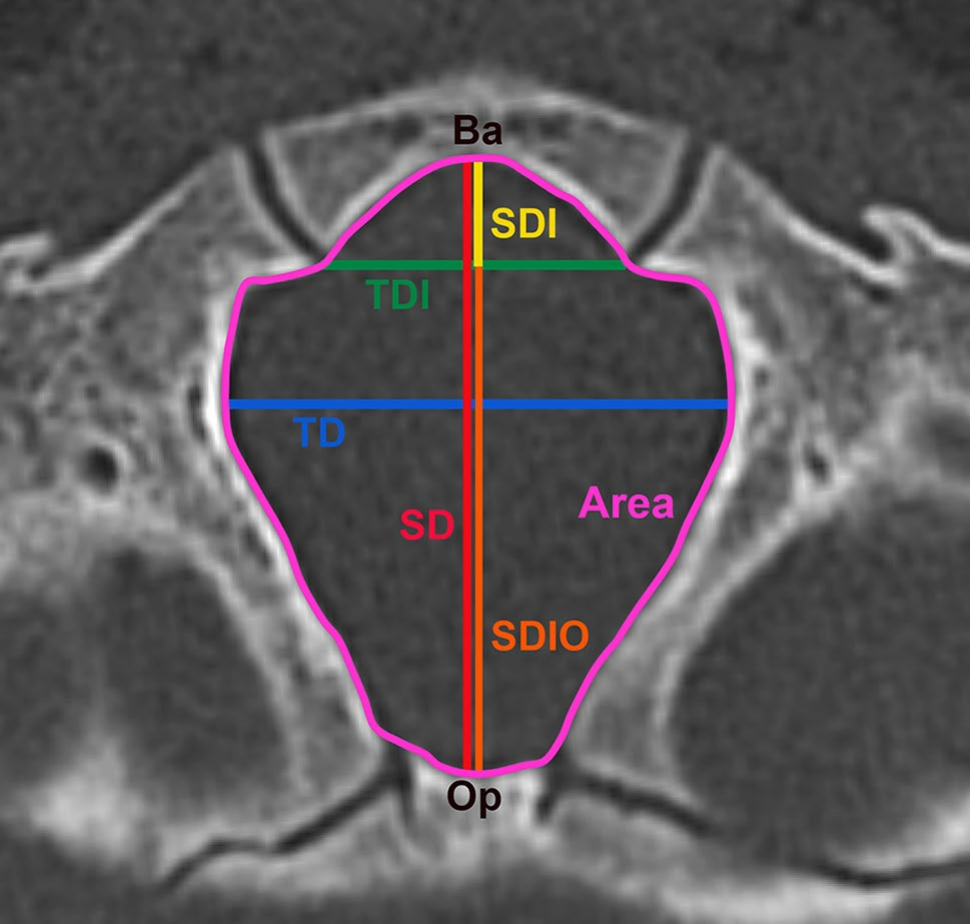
Measurements taken at the level of FM (CT scan, axial cut); Ba, basion; Op, opisthion; area—surface within the purple line
